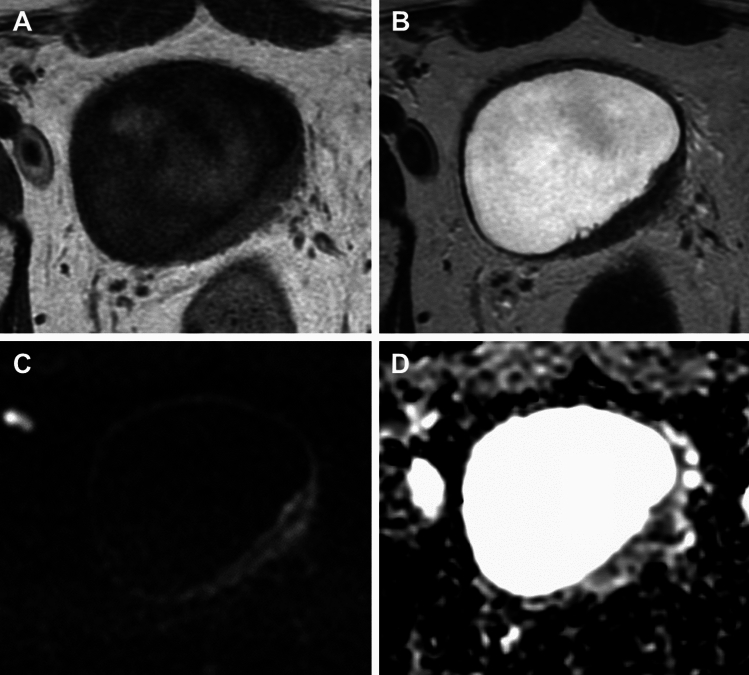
Axial T1WI (A), T2WI (B), DWI (C) and ADC map (D) in a 75-year-old male with urothelial carcinoma who underwent MRI after TURBT shows thickening and perivesical stranding at the left posterolateral bladder wall post-TURBT. Based on the anatomical sequences this finding could be interpreted as T3b disease (A, B), however there was no diffusion restriction to suggest residual/recurrence disease (C, D), the staging was therefore organ-confined disease. Cystectomy showed no residual disease.
